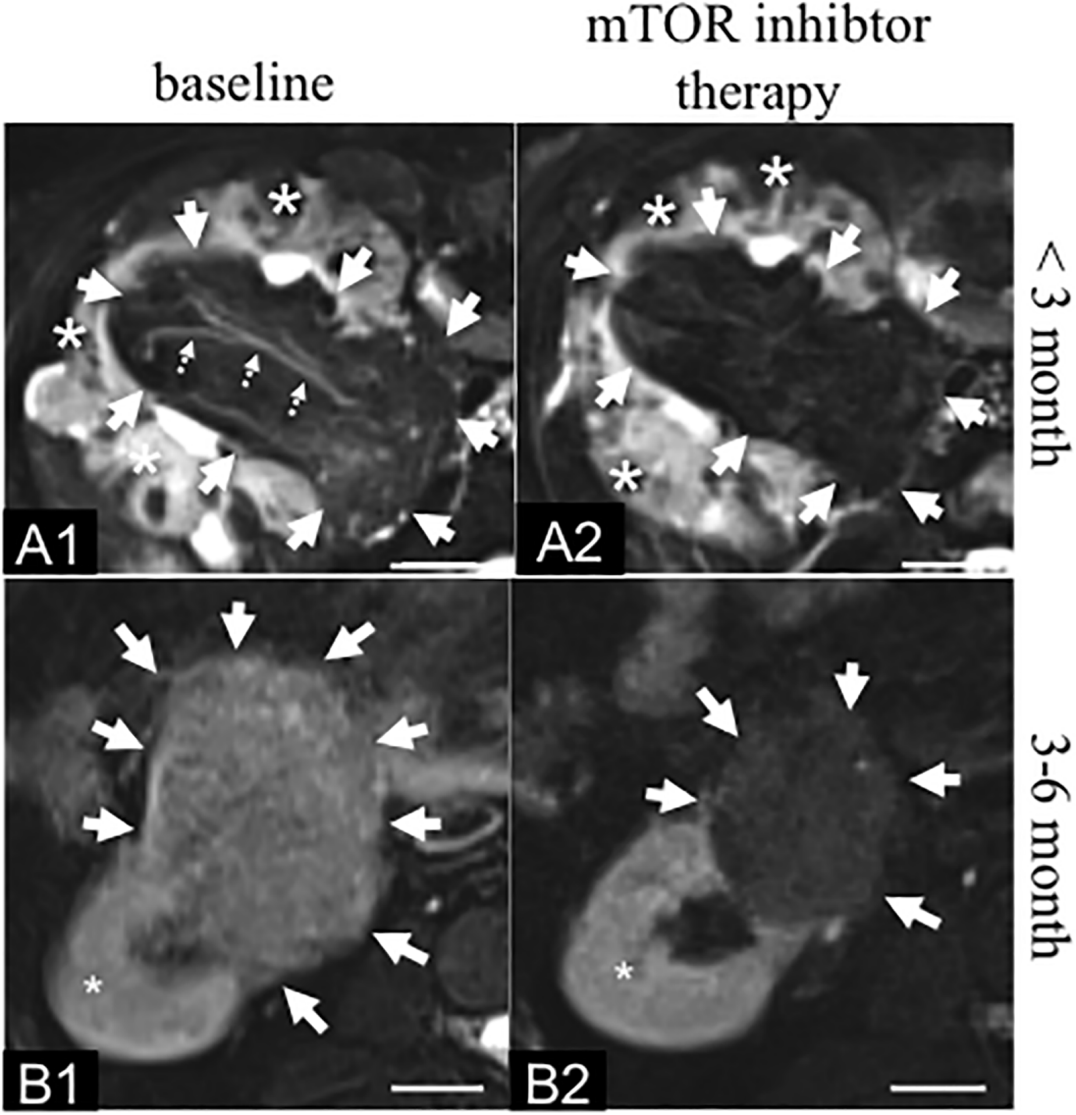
Fatty transformation of angiomyolipoma in TSC patients after initiation of mTOR inhibitor therapy. A: Example of a large AML at the central part of the right kidney in a 33 year-old female TSC patient. A1: At baseline a heterogeneous AML with a mixed-signal on the T2 fat saturated MR sequence (solid arrows) could be visualized. Slow flowing blood in a small vessel (dotted arrows) appears hyperintense on the fat saturated sequence. The unaffected “healthy” kidney tissue is also visualized with a hyperintense signal (*). A2: 2.5 months following the initiation of the mTOR inhibitor therapy a reduction in the overall signal of the AML on the T2 fat saturated sequence can be visualized. The vessel, which was visible at baseline (A1: dotted line) cannot be delineated anymore. Additionally, a reduction of size of the AML can be visualized. This case represents a good example of how difficult it can be to clearly quantify a size reduction in a heterogenous AML following the initiation of mTOR therapy. B: Example of a AML at the caudal part of the right kidney in a 45 year-old male TSC patient. B1: At baseline a heterogeneous relatively bright angiomyolipoma could be visualized on the T2 fat saturated sequence (arrows). The unaffected “healthy” kidney tissue is visualized with a homogenous hyperintense signal on the T2 fat saturated sequence (*). B2: 3 to 6 months following the initiation of the mTOR inhibitor therapy, a clear reduction in the signal on the T2 fat saturated sequence as well as in the size of the angiomyolipoma is visualized. The signal of the healthy kidney tissue does not change following the initiation of the therapy (*). MRI: Magnetic Resonance Imaging. Fatsat: Fat saturated. Scale bar: 1.5 cm.
